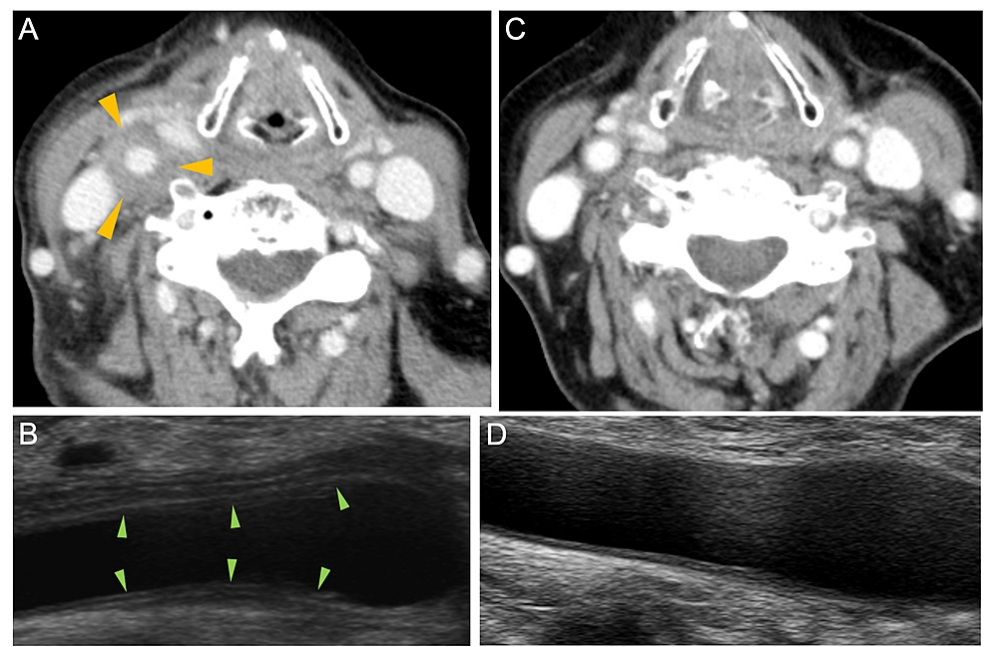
Contrast-enhanced computed tomography and cervical artery ultrasound imaging before (A) and (B) and after (C) and (D) corticosteroid therapy. Contrast-enhanced computed tomography (yellow arrows) and ultrasound imaging (green arrows) revealed wall thickening in the right common carotid artery before treatment. Five weeks after starting treatment, the wall thickening in the right carotid artery had disappeared.
